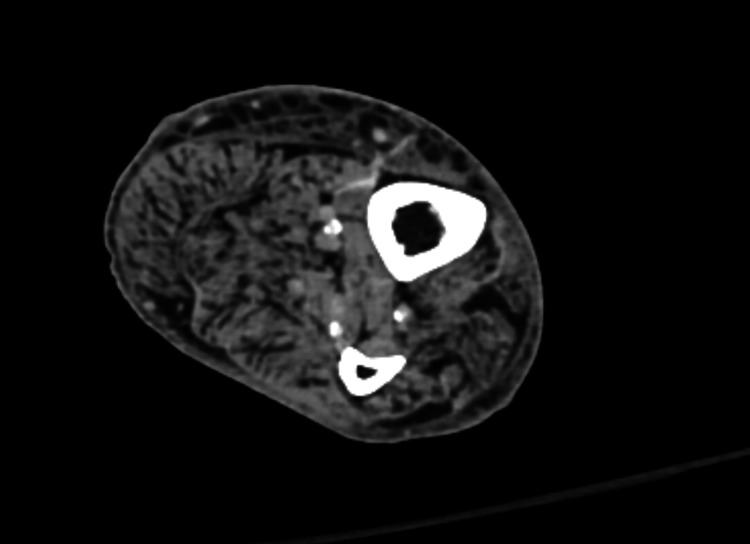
CT of the left lower extremity with contrast showing grossly edematous subcutaneous soft tissues at the distal half of the left leg. No subcutaneous emphysema. No organized fluid collection identified to suggest abscess.
